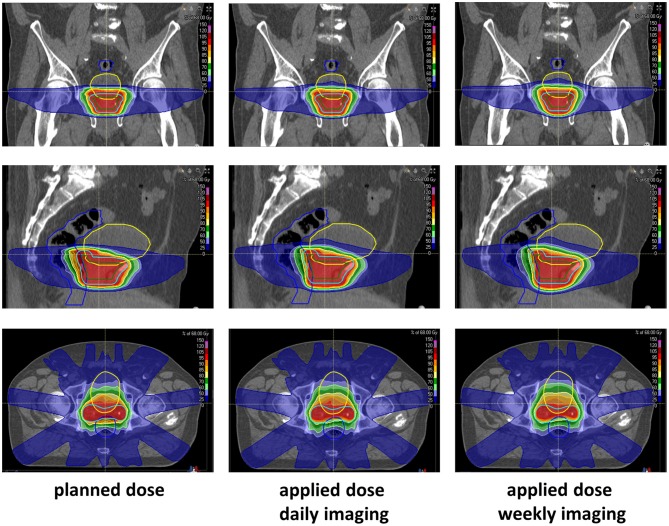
Representative CT imaging demonstrating the planned and accumulated doses based on daily or weekly CT-based repositioning.
